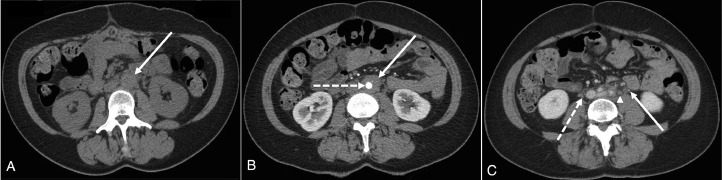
Selected soft tissue window (W:450, L:50) axial computed tomography (CT) slices of a 42-year-old female who presented with left flank pain. (A) Initial noncontrast CT of the kidneys, ureters, and bladder (patient prone) through the level of the kidneys did not demonstrate radiopaque urinary tract calculi. However, subtle low-volume periaortic inflammation (solid white arrow) was identified. The patient was recalled for repeat CT imaging with iodinated contrast. (B) Contrast-enhanced arterial phase imaging at the same level as (A) confirmed circumferential inflammation (solid white arrow) of the contrast opacified aorta (dashed white line) with commensurate narrowing of the aortic caliber from the level of the infrarenal aorta to the aortic bifurcation. There was no aortic ectasia or aneurysmal dilatation. The caliber of the celiac axis, superior mesenteric, and both renal arteries were normal with no periarteriolar inflammation. (C) Portal venous phase imaging at the level of the proximal iliac arteries (inferior to the aortic bifurcation) demonstrated periaortic inflammation extending around the left ureter (solid white arrow; opacified with contrast) which may have been the cause of the patient's left flank pain. An adjacent left para-iliac subcentimeter lymph node (white arrowhead) was also identified alongside a small amount of inflammatory change around the right ureter (dashed white arrow). The appearances were consistent with an isolated periaortitis. A serum erythrocyte sedimentation rate and rheumatology opinion were advised.
